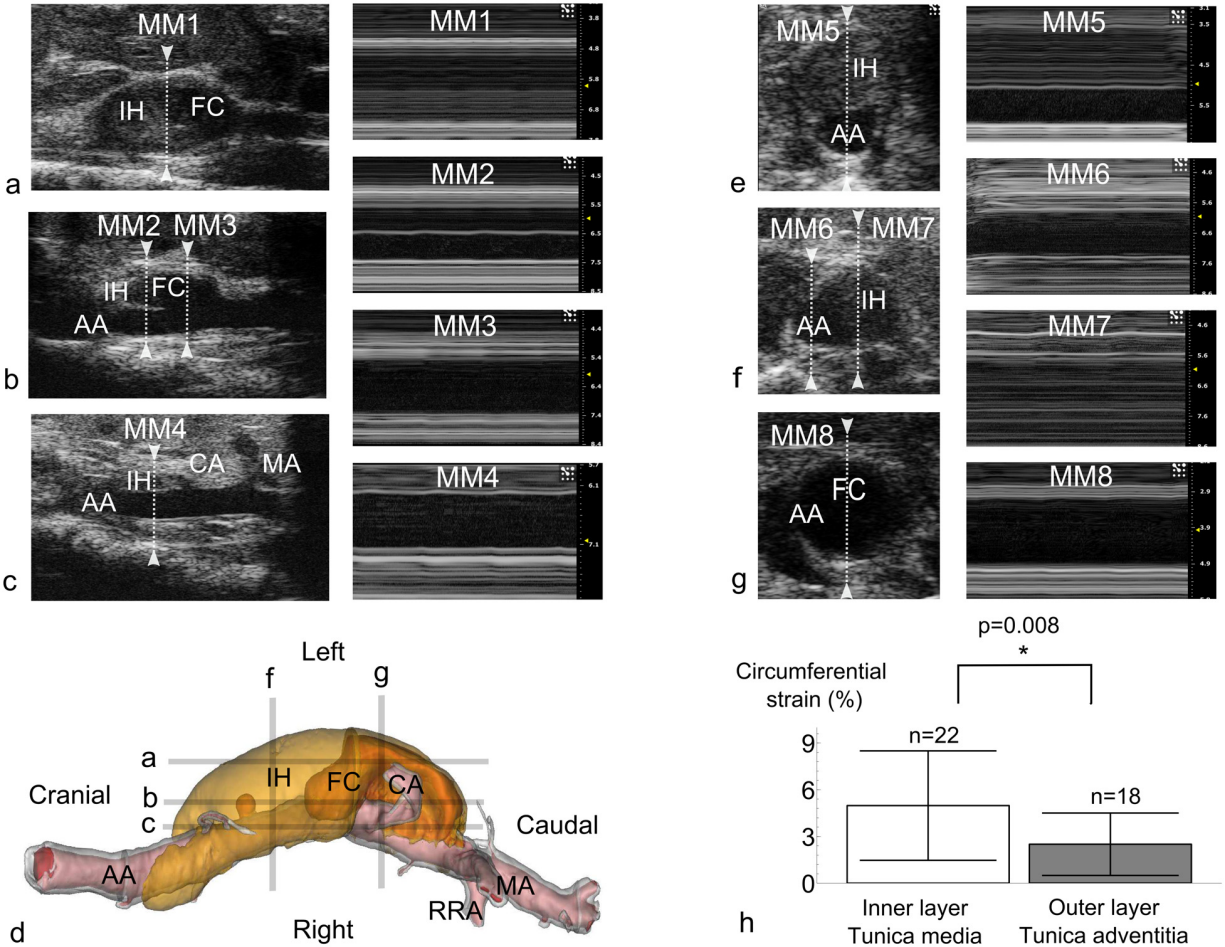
Diameter assessment in dissecting AAAs. a. Long axis view taken at the level of the intramural hematoma and false channel, where the abdominal aorta is not visible. b. Long axis view taken at the level of the tear in the tunica media, showing a dissecting flap. The image in MM2 shows how the inner layer (tunica media) is more distensible than the dissected outer layer (tunica adventitia), while the tunica media is not visible when measuring at MM3. c. Long axis view taken at the level of the aortic lumen, the false channel is not visible, and the intramural hematoma seems much smaller than in reality. d. 3D visualization of dissecting AAA based on PCXTM scans. Image taken from [42] for visualization purposes. The ultrasound images shown in panels a-c (LA MMode) and d-f (SA MMode) do not correspond to the actual 3D geometry shown on the top panels, but were taken at locations that were similar to the indicated lines. IH: Intramural Hematoma. FC: False Channel. CA: Celiac Artery. MA: Mesenteric Artery. RRA: Right Renal Artery. AA: Abdominal Aorta. e. Short axis view showing an intramural hematoma that is located on the anterior side of the aortic lumen. f. Short axis view showing an intramural hematoma that is located on the left side of the abdominal aorta. g. Short axis view taken at the level of the false channel. h. Short axis MMode circumferential strain in matched pairs (n = 19) where both a dissected adventitia and tunica media could be measured simultaneously (cfr. MM2). The inner, medial layer is more distensible than the outer, adventitial layer. Since the pulse wave travels over the innermost layer, this will influence the PWV value obtained with transit time methods.
